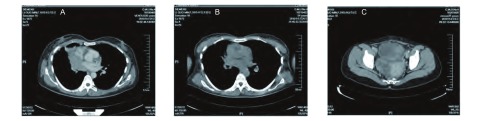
患者2的影像学特点。A：化疗5周期后（即放疗前）肺CT；B：放疗后2月肺CT；C：盆腔CT。 CT imaging of case two, 34-year-old woman with SCCL presented with bilateral ovarian metastases; A: lung imaging on CT after five circles of chemotherapy; B: lung imaging on CT after two months of radiotherapy; C: pelvic cavity imaging on CT.
